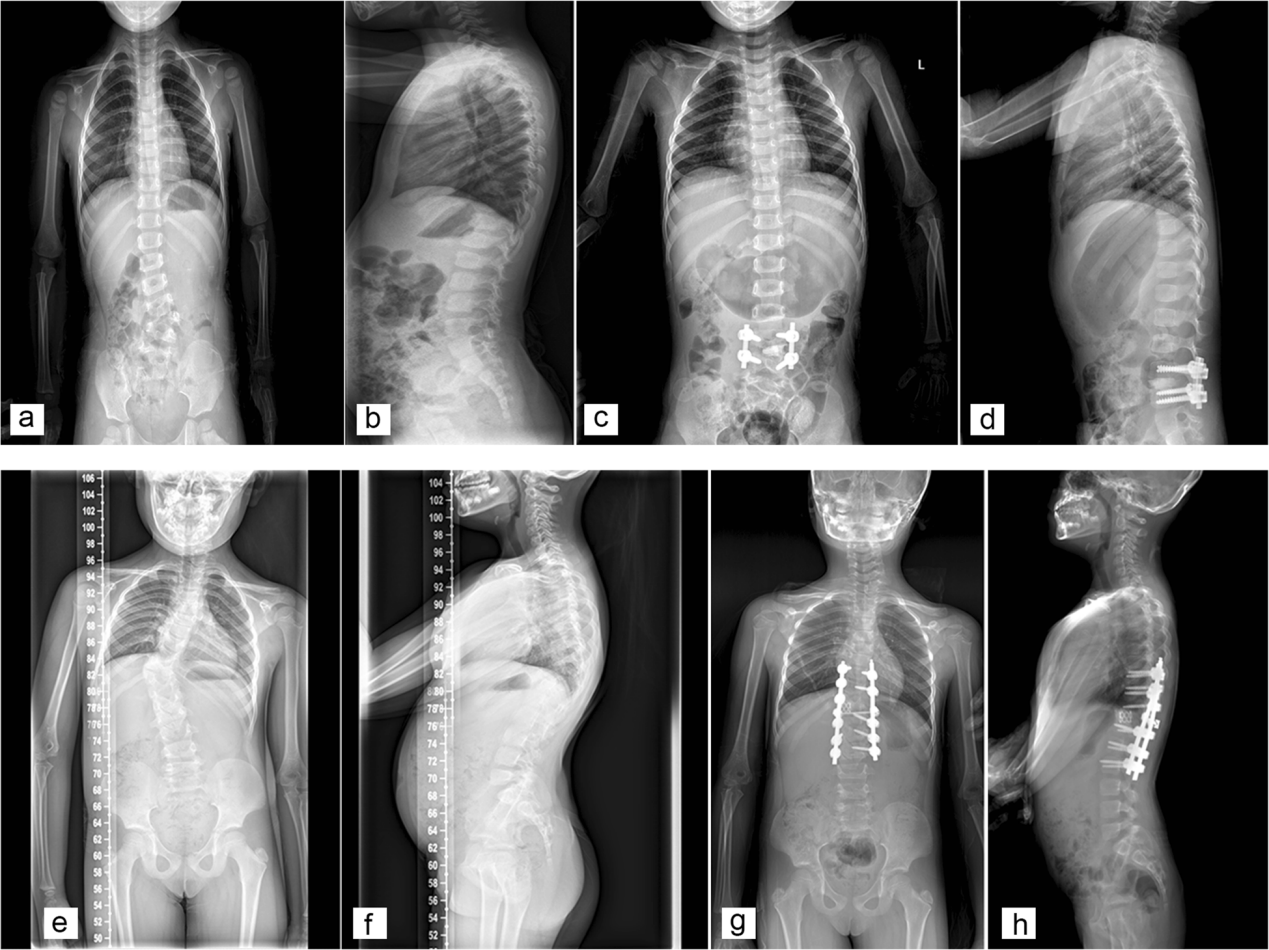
Preoperative and postoperative radiographs of two patients. a, b, c, d:a 2-year-old girl with a fully segmented hemivertebra at L4/5. e, f, g, h:a 7-year-old girl with a hemivertebra at T11
